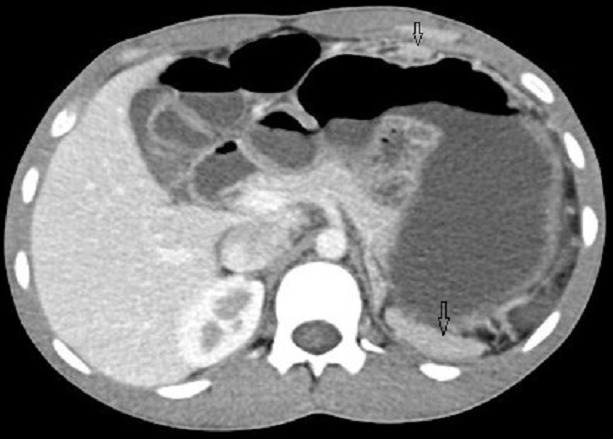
Axial image of contrast enhanced CT showing homogenously enhancing omental and left lumbar lesions (arrows)
